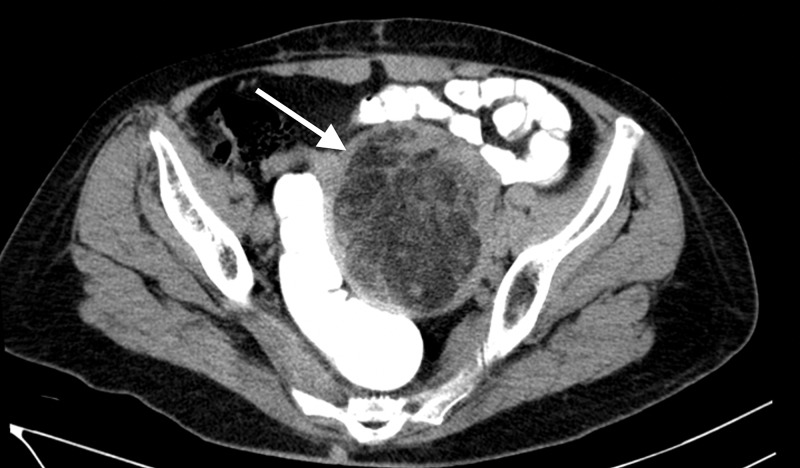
Axial CT image through the pelvis, with rectal contrast, revealing a circumscribed pelvic mass containing fat and soft tissue density arising from the uterus (white arrow).
